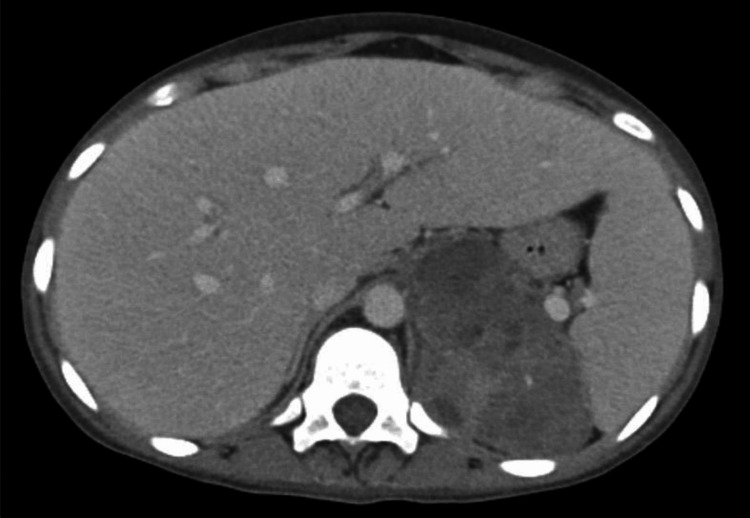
Axial enhanced CT scan showing a large heterogeneous left adrenal mass.
